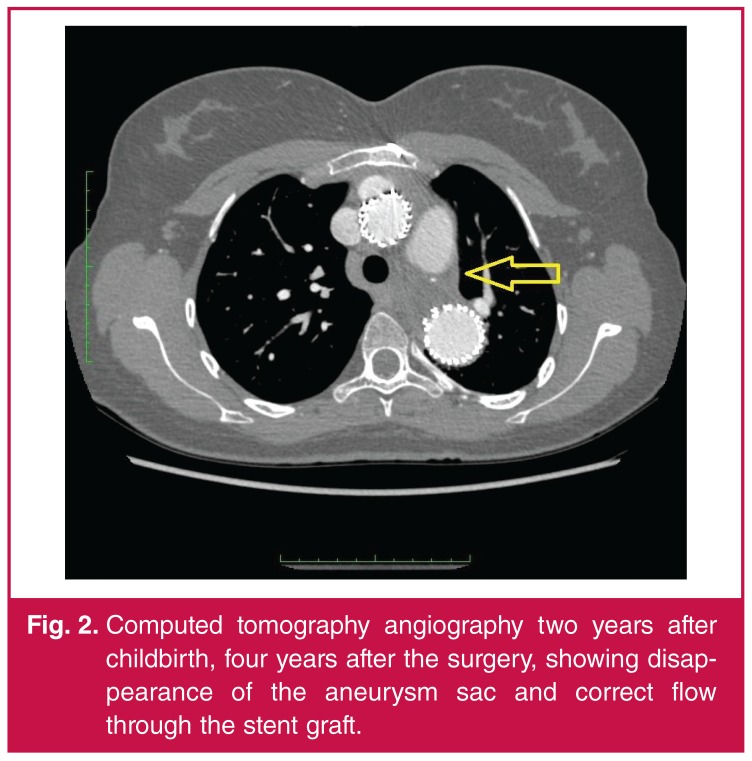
Computed tomography angiography two years after childbirth, four years after the surgery, showing disappearance of the aneurysm sac and correct flow through the stent graft.
